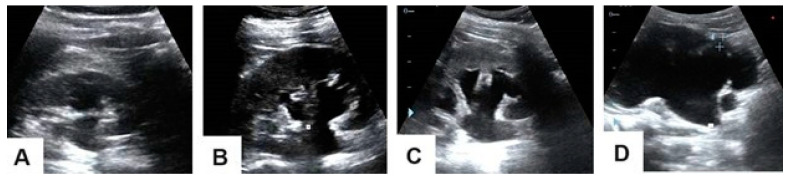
Selected ultrasonography images of hydronephrosis grades showing (A) Grade 1 with renal pelvis dilatation, (B) Grade 2 with mild caliceal dilatation, (C) Grade 3 with severe caliceal dilatation, and (D) Grade 4 with parenchymal atrophy.
